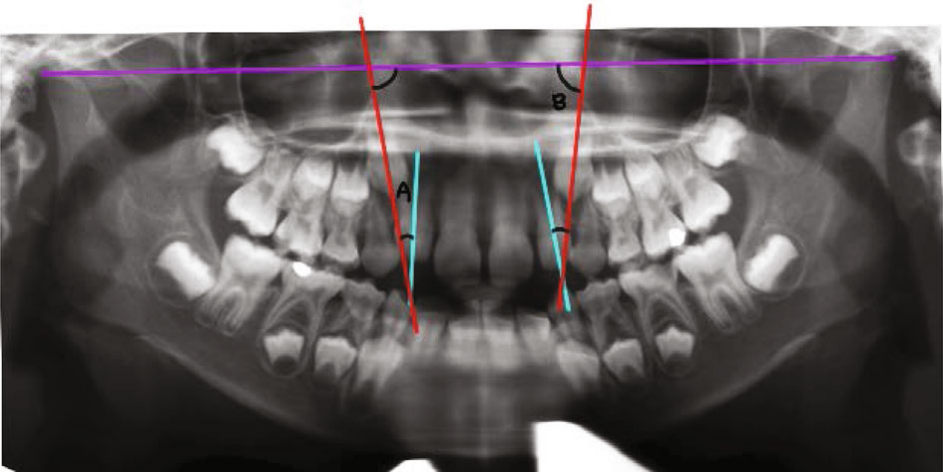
Showing parameters 1 and 2 traced on a panoramic radiograph.
